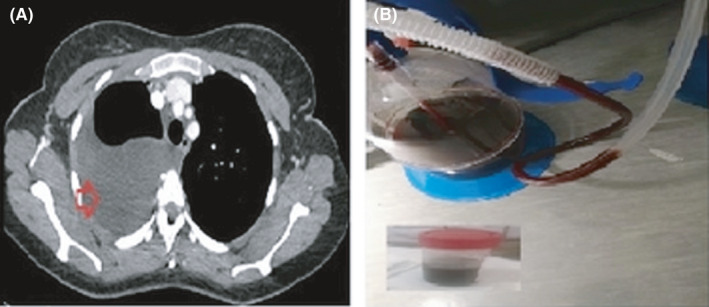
A, CT chest showing large right‐sided free‐floating effusion (red arrow). B, Chest tube with dark‐brown (Chocolate) appearance of the pleural fluid
